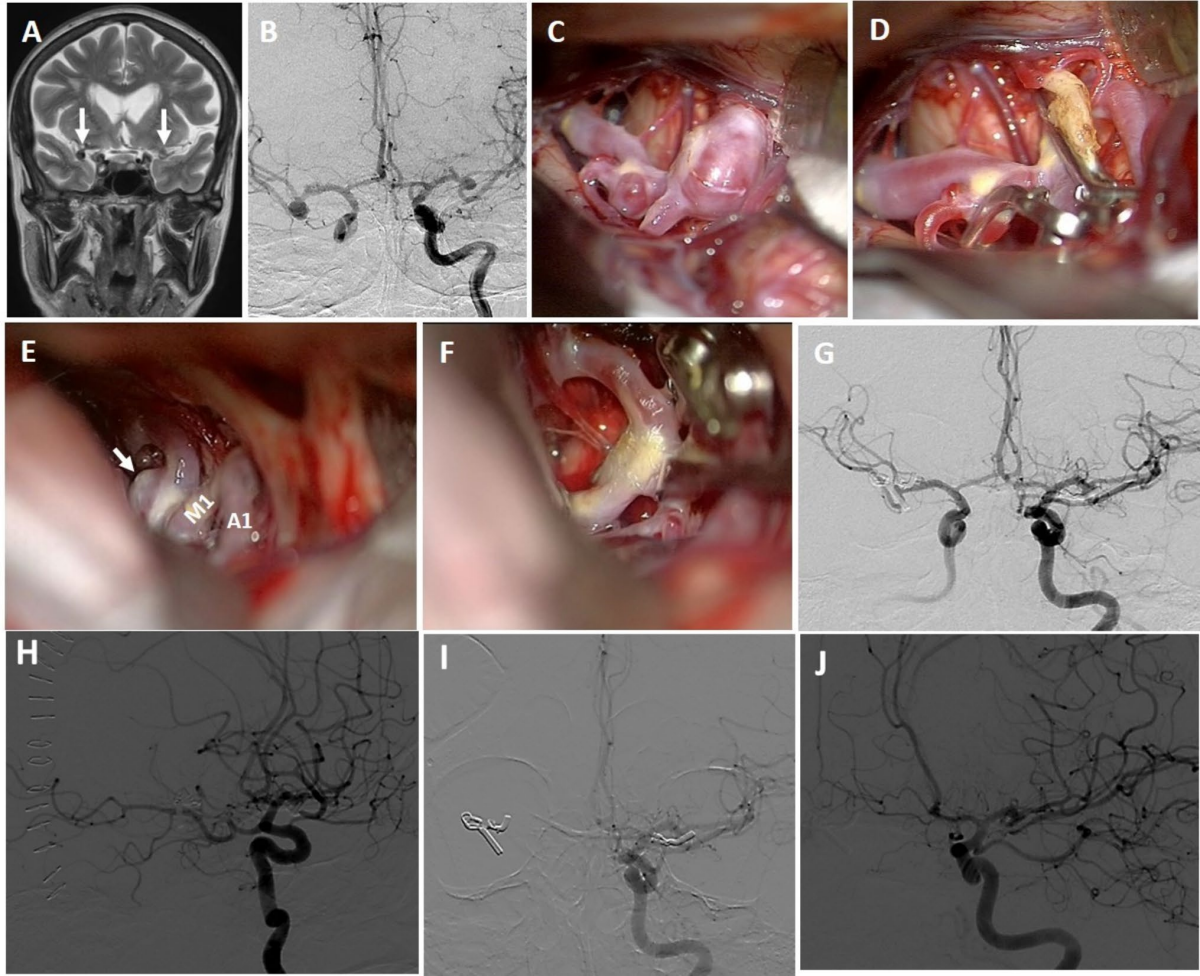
A T2-weighted MR image shows bilateral aneurysms (arrows), B DSA demonstrates three aneurysms: in the right MCA bifurcation and M1 segment and in the left MCA bifurcation, C At first, both aneurysms in the right MCA were explored, D were clipped, E and then right A1 and left A1 segments were followed and the left ICA bifurcation was reached. Tortuous left M1 segment was followed towards the bifurcation and a posterior (lateral) projection aneurysm was seen. F The perforating arteries at the bifurcation were dissected and the aneurysm was closed with a slightly bent clip passing under the inferior trunk. G, H, I, J Postoperative DSA confirms occlusion of three aneurysms
